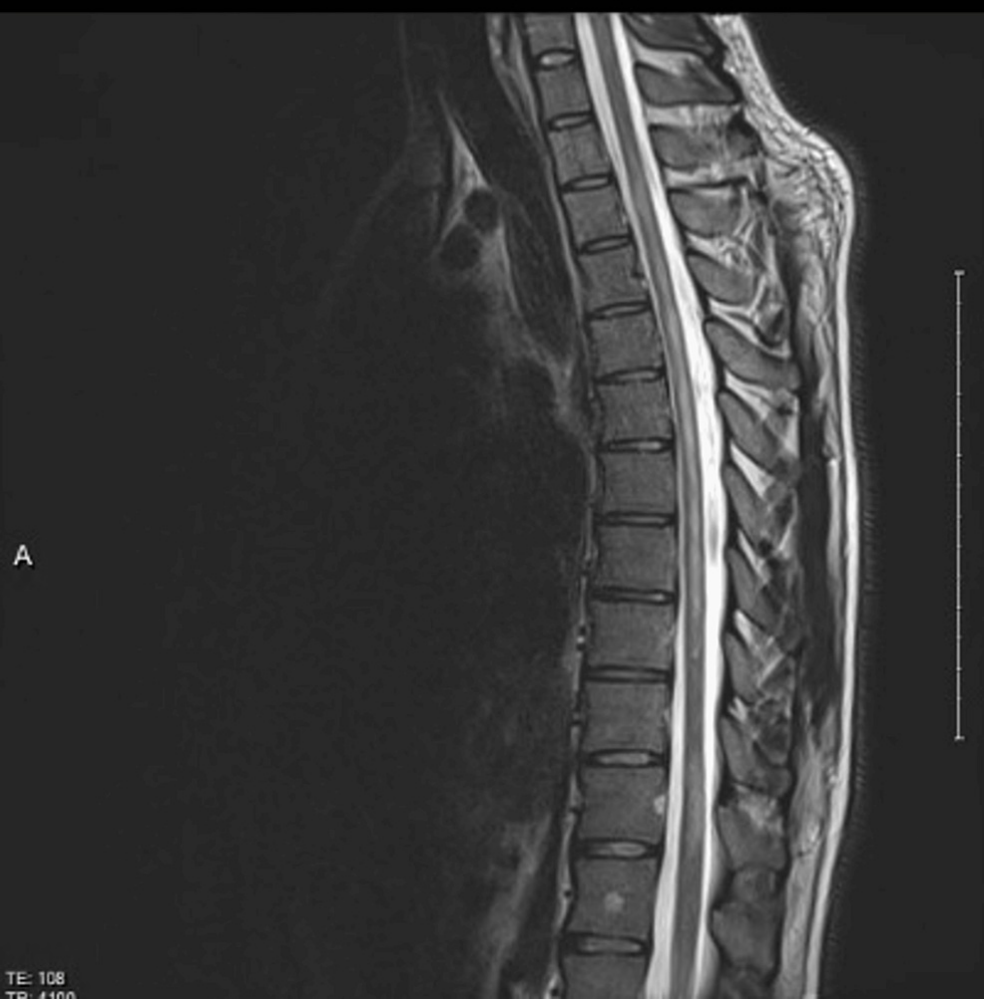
Follow-up MRI of the thoracic spine with contrast (two weeks after the initial MRI)
